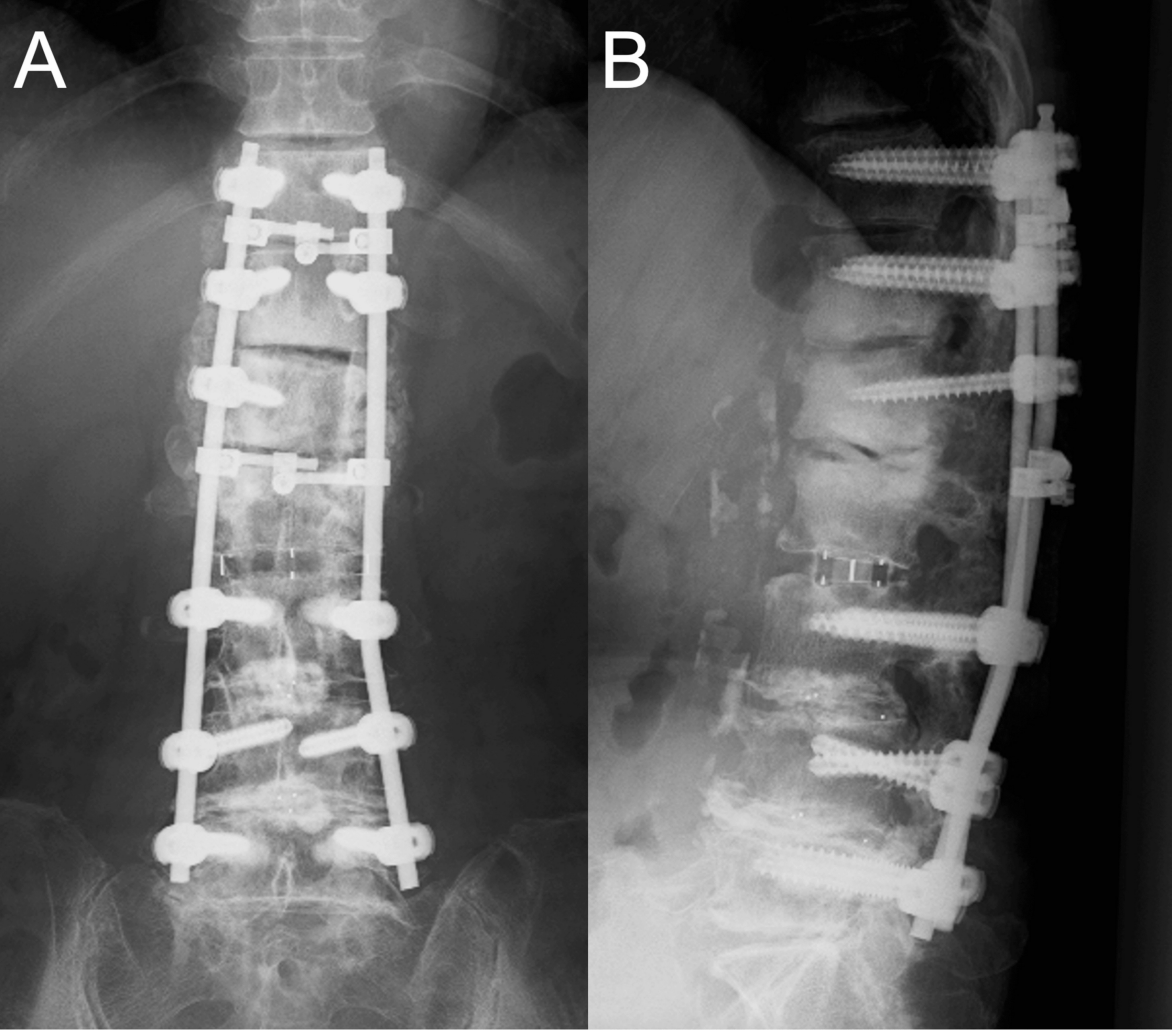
Postoperative radiography images. (A) Anteroposterior view. (B) Lateral neutral view.
